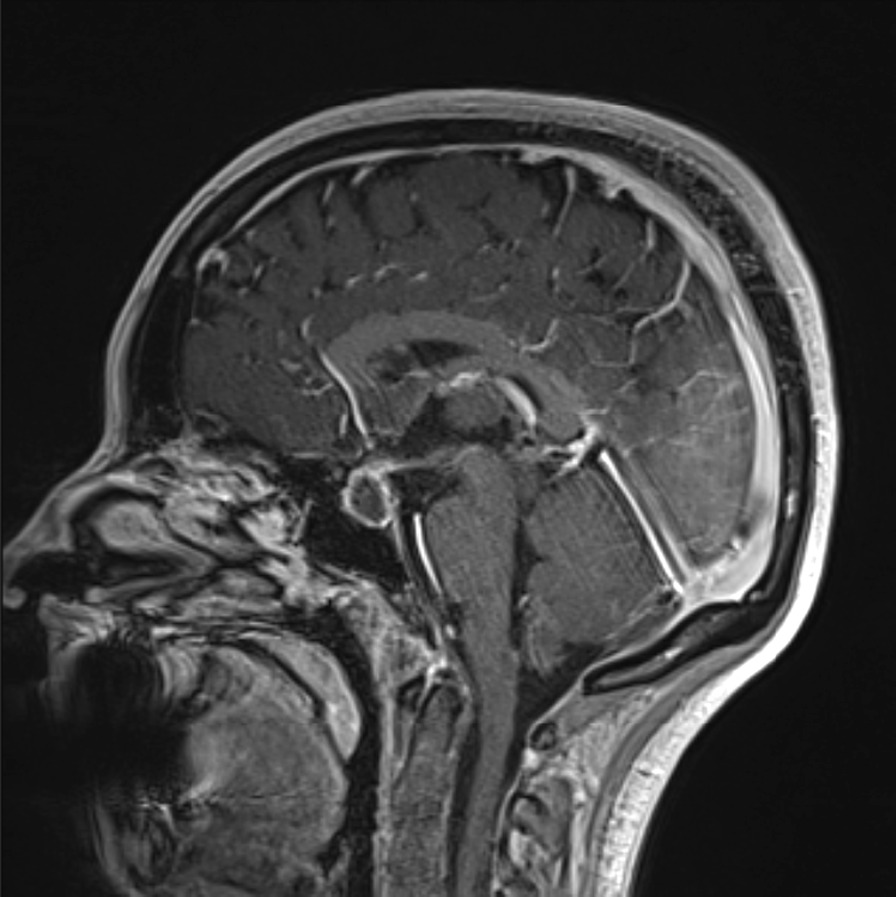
Post-operative MRI brain scan post-gadolinium T1 sequence showing a pituitary mass with peripheral contrast enhancement
